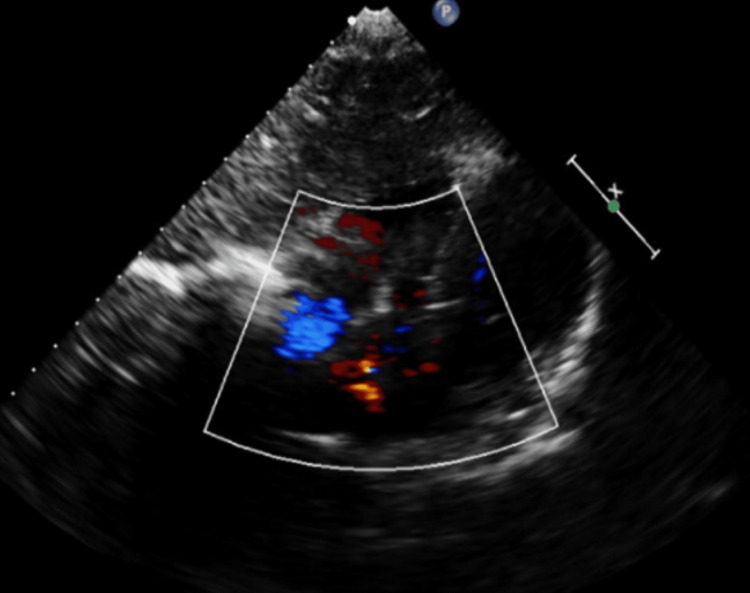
Color Doppler ultrasonography of the right parotid region demonstrating low-flow vascularity consistent with a venolymphatic malformation.
